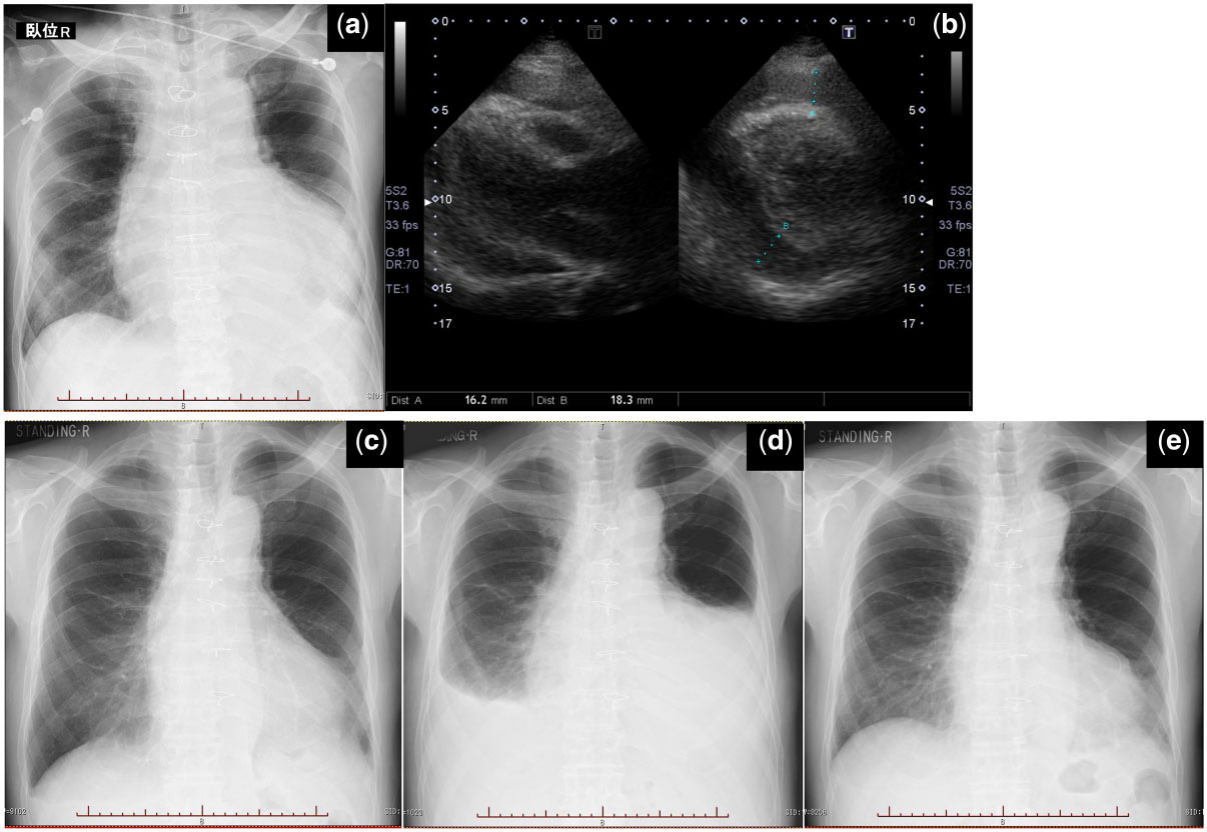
Chest radiography and echocardiography on initial admission (a and b), at discharge (c), on readmission (d) and at 3-year follow-up (e).
